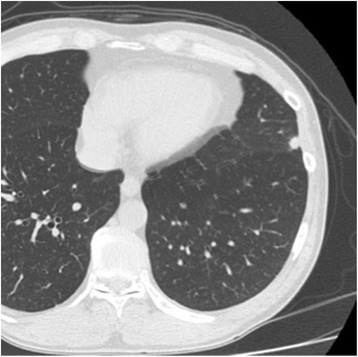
Computed tomography. A small nodule was found in the lingular segment
